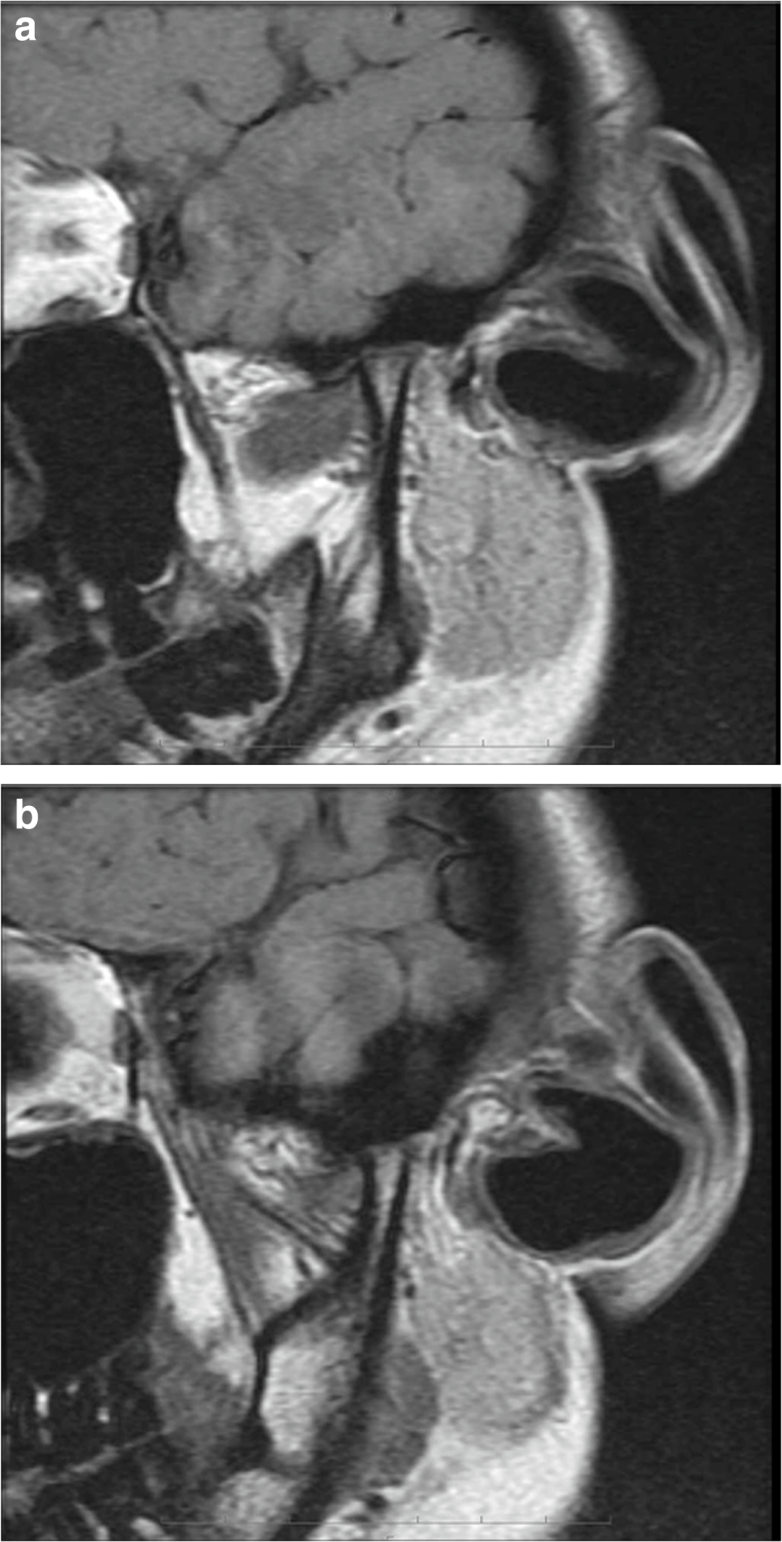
Pretreatment magnetic resonance imaging. a Right side; b left side
